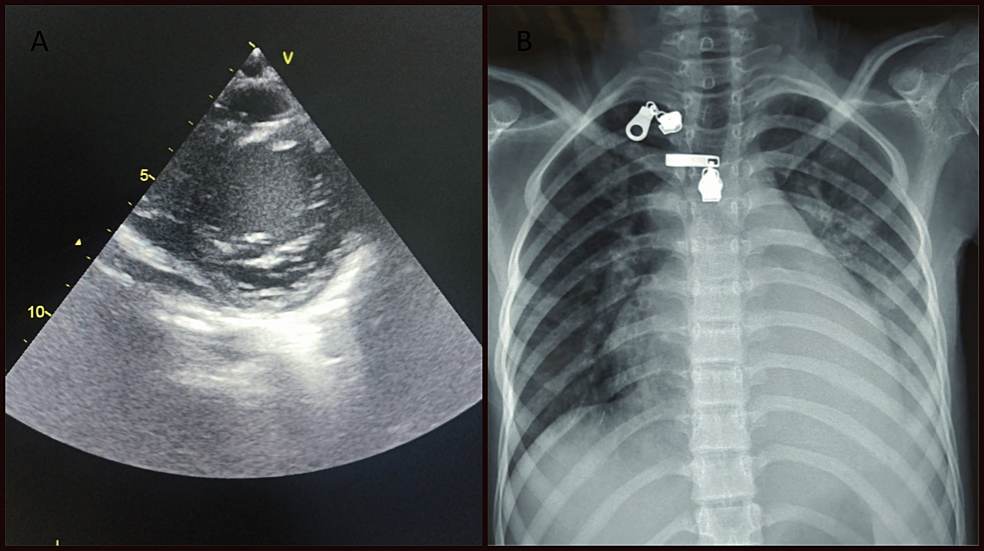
Radiological signs in our case, A: pericarditis on echocardiography; B: pleurisy on chest X-ray
